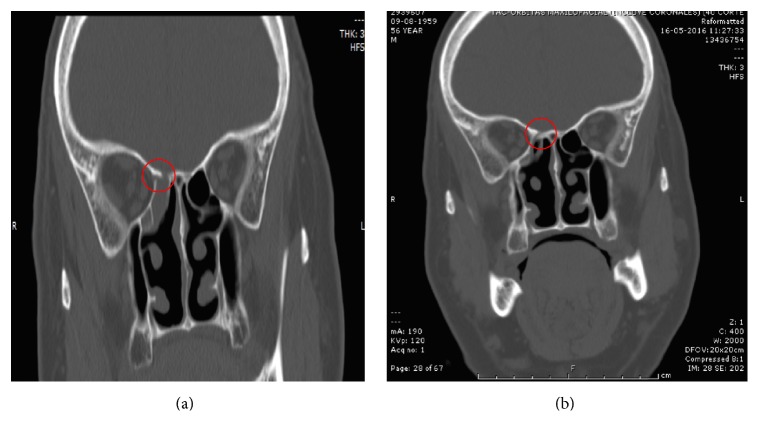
Case 1. (a) Preoperative CT scan showing a CSF leak in the right cribriform plate. (b) Seven-month postoperative CT showing a less dense bony closure of the surgical defect.
